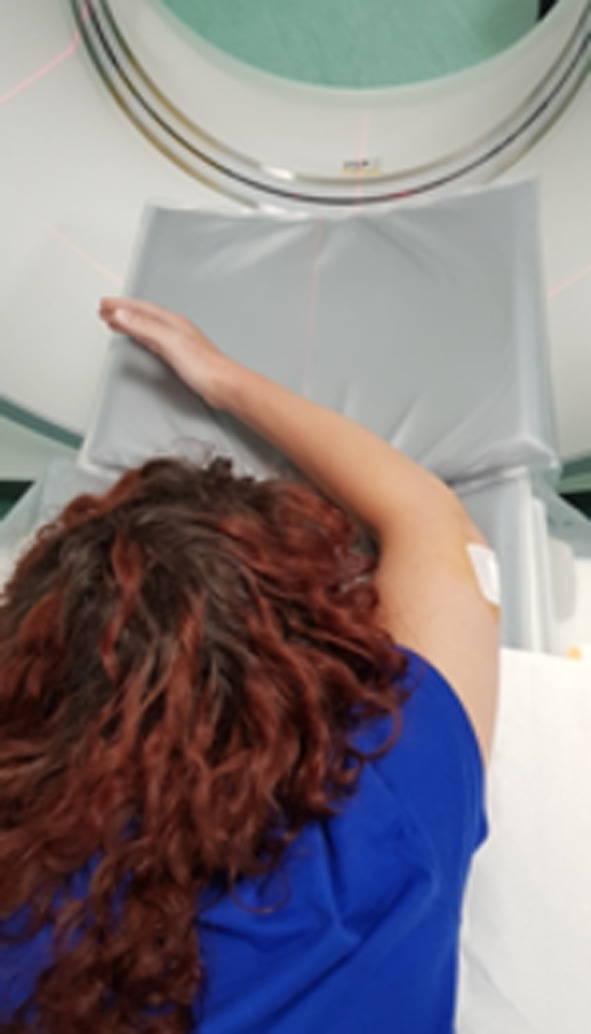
The patient is positioned prone on the CT scanning table, with the affected upper limb elevated over the head and with a 45° flexion of the elbow
